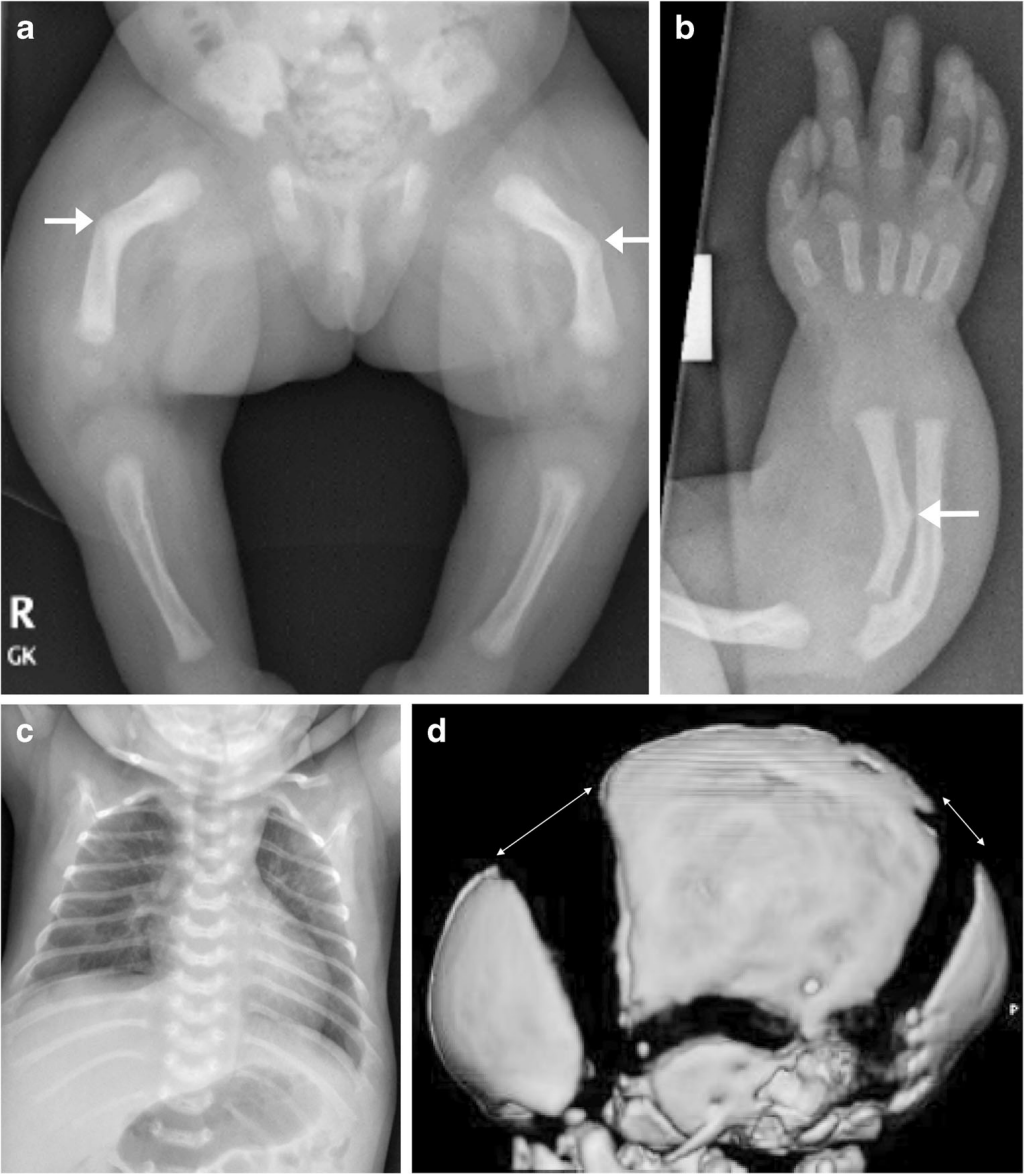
Imaging features of hypophosphatasia in a 3-month-old girl. a-c Anteroposterior radiographs show short bowed femora (*arrows*) (a), short bowed radius and ulna with spurred radius (*arrow*) of the right forearm (b), and slender, poorly ossified ribs in the chest (c). d Three-dimensional CT reconstruction in the same child shows wide sutures and fontanelles (*double-headed arrows*). [Images reproduced with permission from Radcliffe Publishing [38], pages 375–376, case 12, images 12n, 12p, 12q, and 12s]
